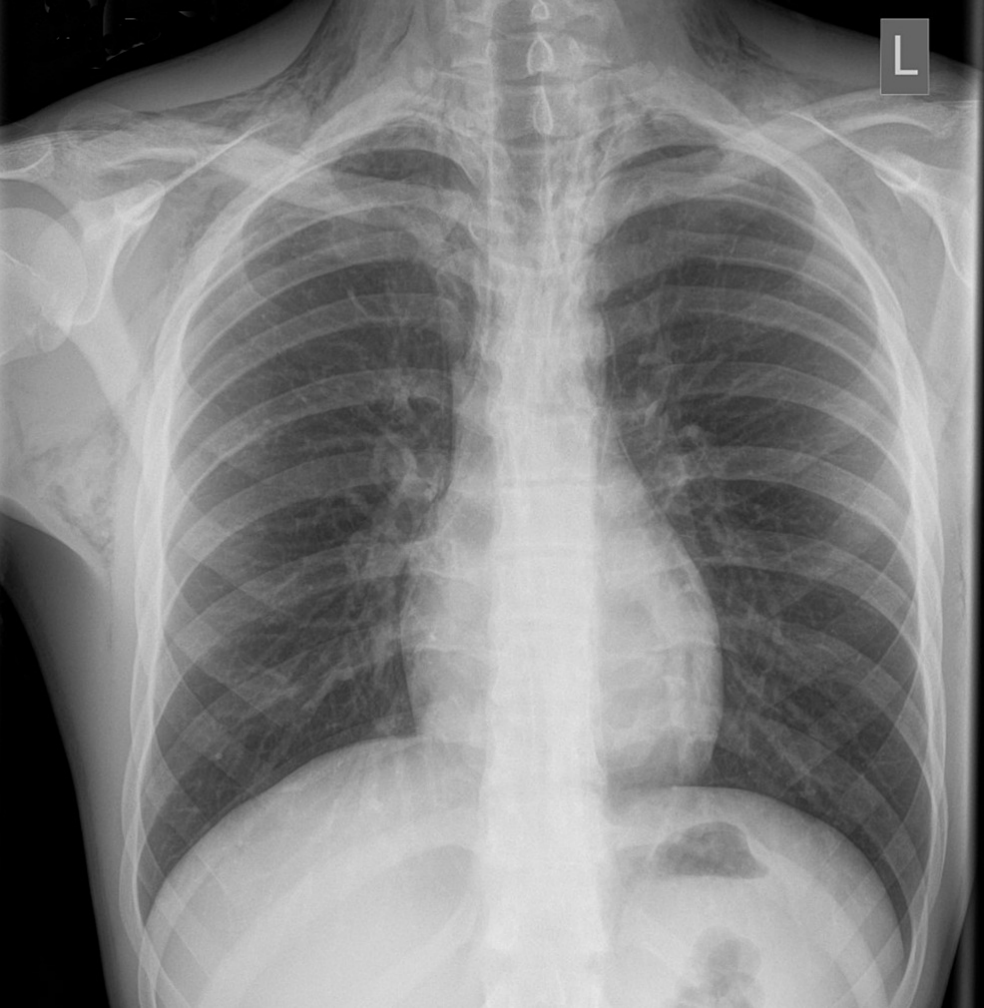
Chest X-ray on day 1 showing neck soft tissue emphysema and pneumomediastinum.
